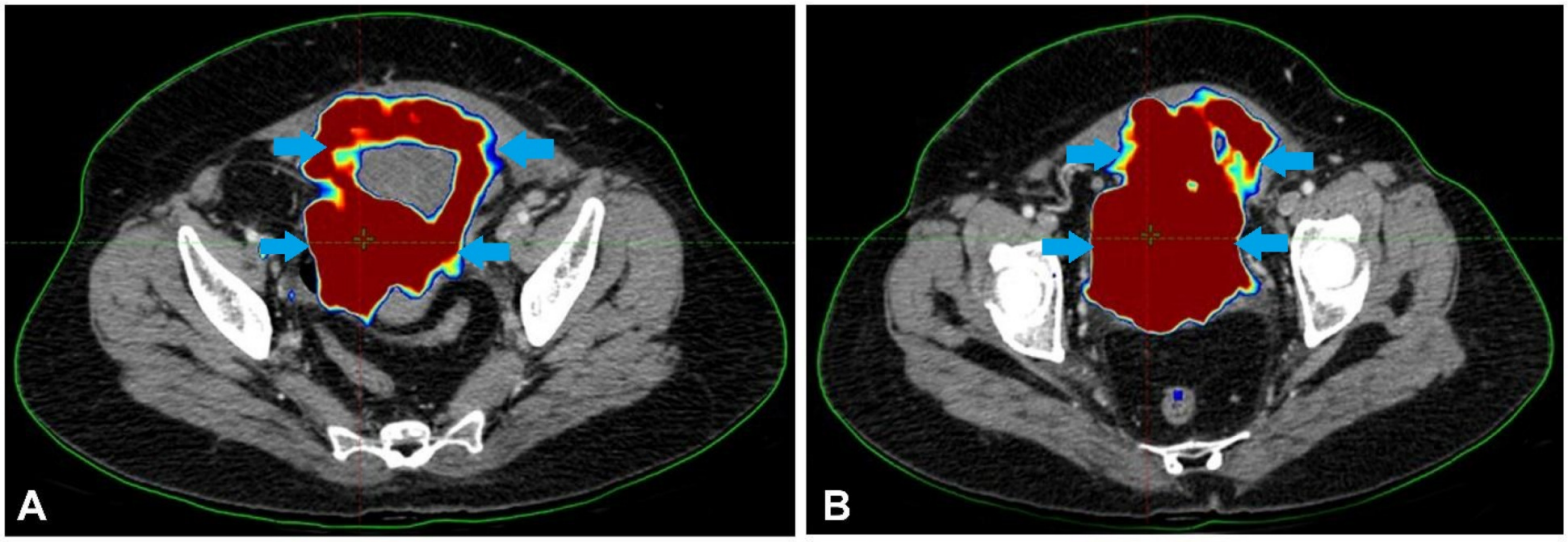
The initial PET-CT scan showed an irregular soft tissue mass at the bladder apex with areas of high FDG uptake, indicating active malignancy.
